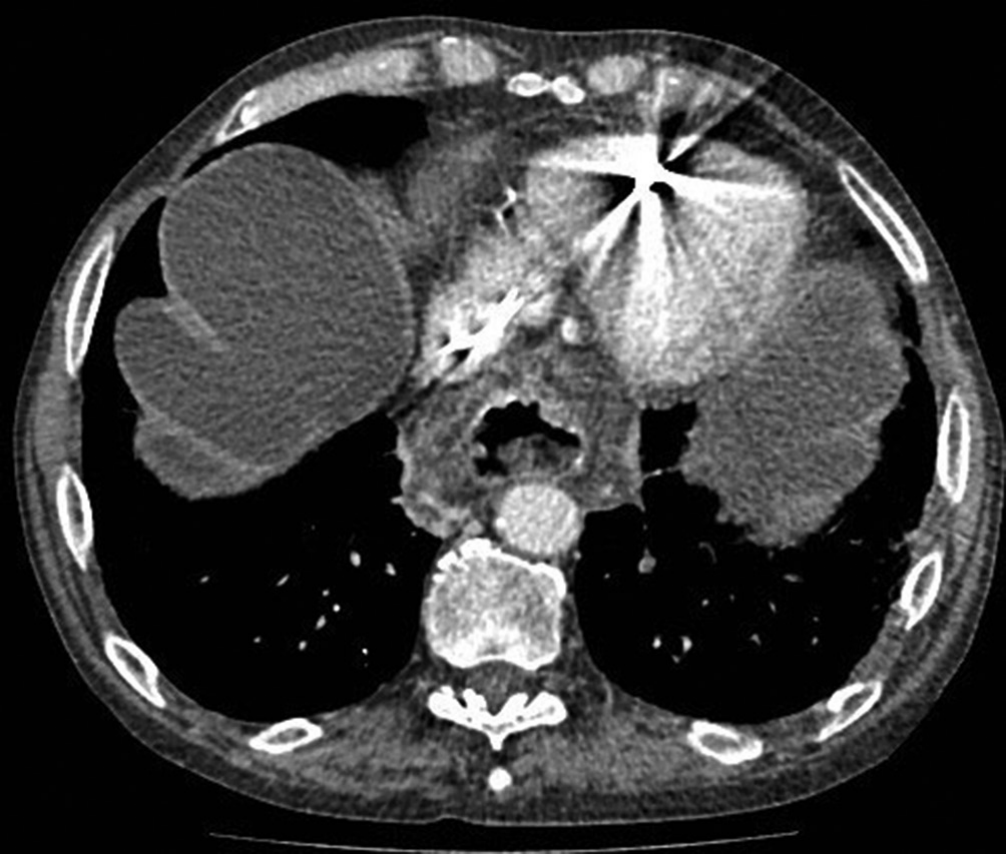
CT abdomen and pelvis March 2017 demonstrating presence of ascites and hiatus hernia.
